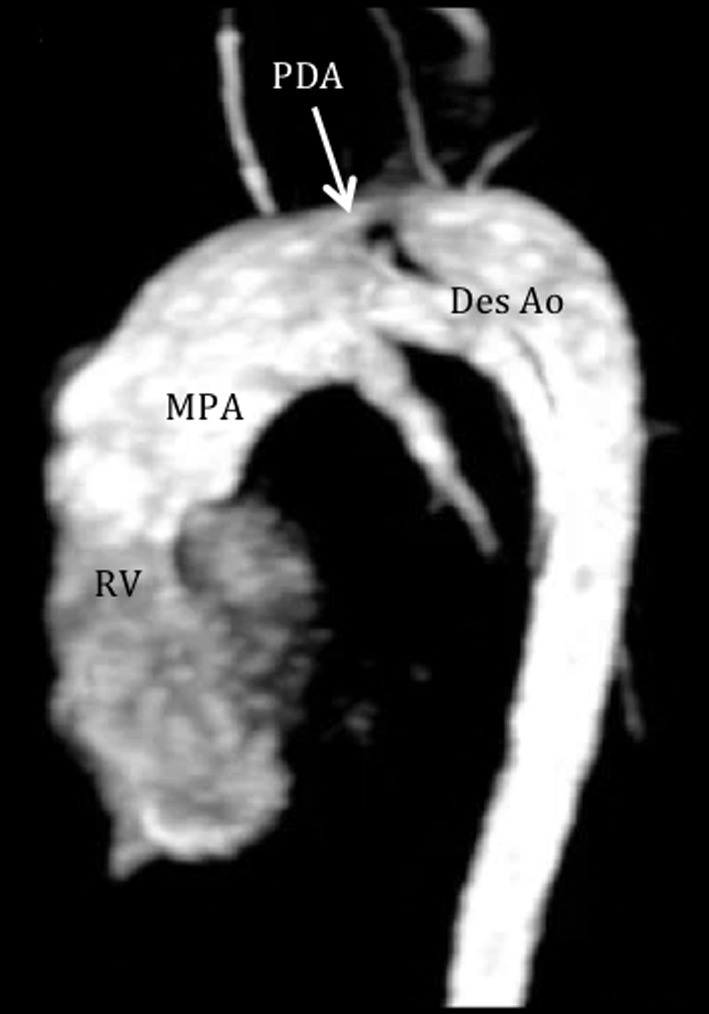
Cardiac MRI demonstrates a patent ductus arteriosus. Pulmonary angiogram shows flow from the pulmonary artery to the descending aorta through a patent ductus (arrow), measuring 8 mm wide and 15 mm in length. RV: right ventricle; MPA: main pulmonary artery; PDA: patent ductus arteriosus; Des Ao: descending aorta.
